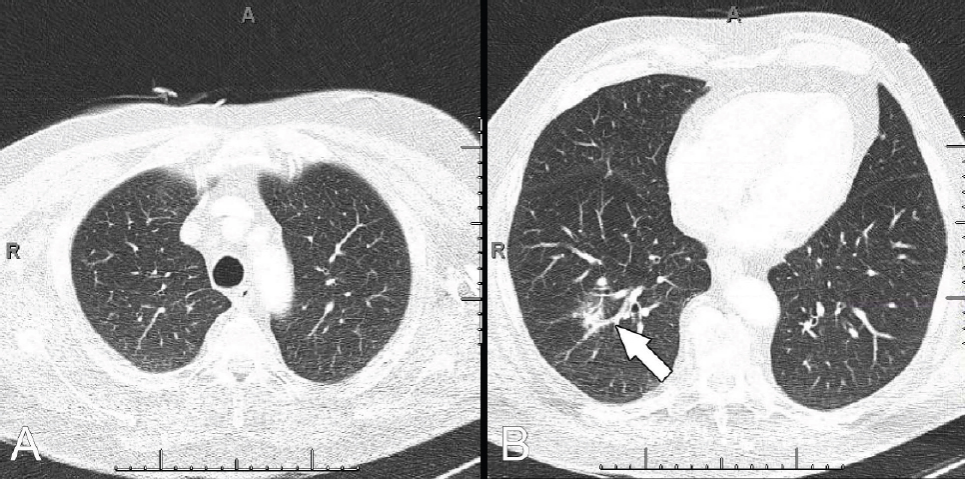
Lung-window chest CT of a stroke CT angiography in a patient with an unsuspicious upper-chest exam (A) and opacifications in the lower parts (B: arrow) (CO-RADS 3).
